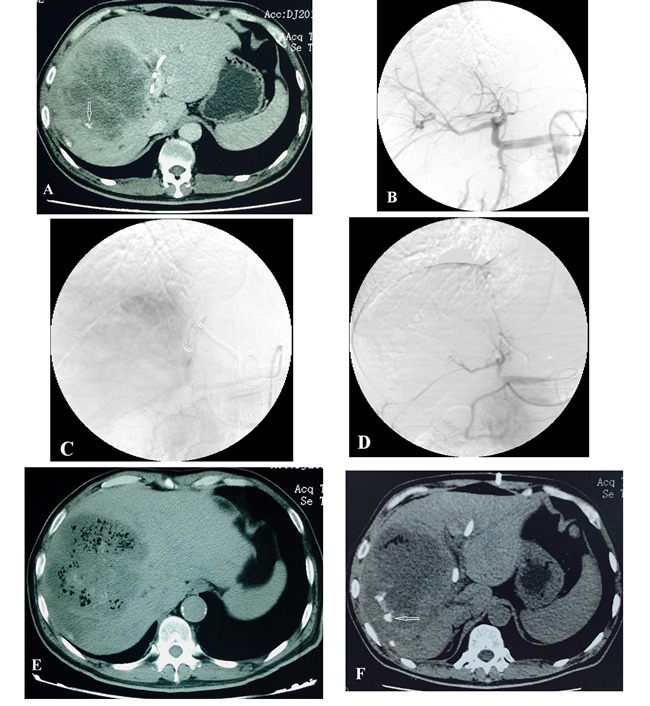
Male patient, 54 years old, with hepatocellular cholangiocarcinoma CT scan shows a hypovascular tumor. The patient had undergone PTCD and TACE (with 1 week interval) 1 month earlier. At review, there was increase in the size of the tumor. Microspheres TAE therapy was applied immediately. A. CT scan shows lesions located in the right lobe of the liver before TAE, poor blood supply, and a small amount of lipiodol deposited around the edge of lesion (white arrow). B. C. Hepatic artery angiography before embolization, shows that the blood supply is not rich. D. After embolization, the tumor blood vessels have disappeared, and the main and secondary branches of the hepatic artery are visible. E. CT plain scan 1 week after embolization shows visible tumor necrosis; many bubbles can be seen. F. Three months after embolization, most lesions have undergone necrosis; a small amount of gas and a little lipiodol deposition can be seen at the edges of the lesions (white arrow).
